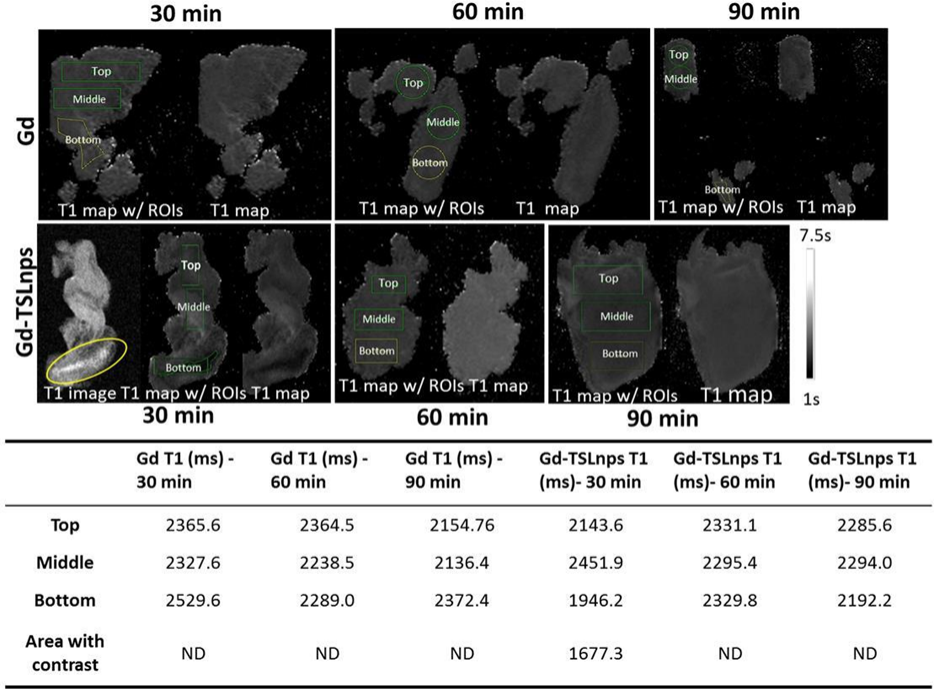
Ex vivo* MRI scan. T_1_ maps of *ex vivo* tumors acquired at 30, 60 and 90 min after i.p. injection of free Gd and Gd-TSLnps. On the left of each sample, the ROI placement for T_1_ extraction is shown. The right full T_1_ map is shown. The 30-min Gd-TSLnps sample showing a region with increased T_1_ contrast also has the magnitude image included. ND = not detected
